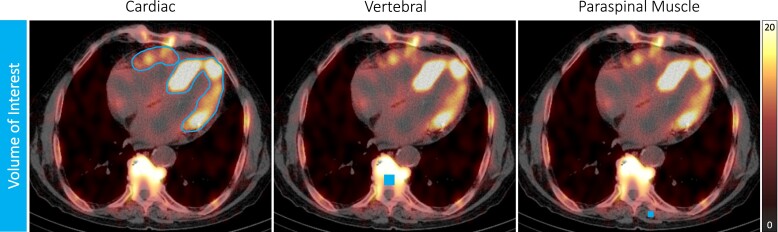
SPECT/CT and volume of interest. SPECT/CT ^99m^Tc-DPD acquisition of a representative treatment-naïve patient with wild-type ATTR-CM showing the VOI for each tissue (VOI cardiac: automatically generated using a threshold-based method, VOI vertebral: 2.92 mL, VOI paraspinal muscle: 1.19 mL) placed to quantify the SUV.
